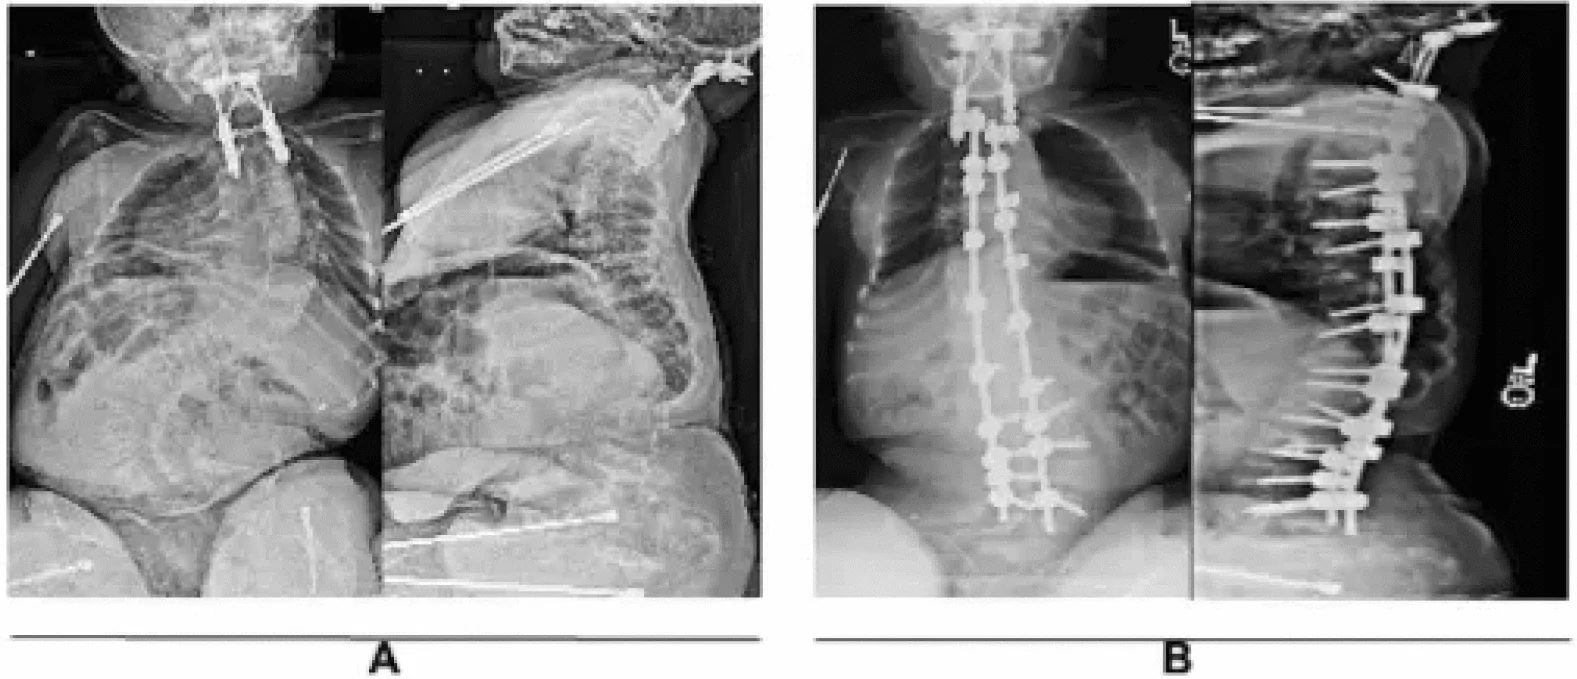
A Preoperative radiographs of a 14-year-old female with type IV osteogenesis imperfecta (OI) and history of prior cervical spinal fusion showing double major curvatures of 112° and 75°. Preoperative measurements included apical vertebral translation (AVT) of 92 mm, thoracic kyphosis of − 38°, thoracolumbar kyphosis of 31°, and lumbar lordosis of 75°. Preoperative SRS-22 total score was 3.0 (pain 2.8, function 3.3, self-image 2.4, mental health 3.4, satisfaction 3.0), CP CHILD total score was 52 (activities of daily living 52, positioning/transferring/mobility 45, comfort and emotions 40, communication and social interaction 55, health 50), and PROMIS T-scores were peer relationships 12, fatigue 25, pain interference 28, and physical function mobility 15. B 2-year postoperative radiographs following posterior spinal fusion with segmental pedicle screw instrumentation. The proximal construct was connected to the existing cervical hardware, while cement augmentation was performed at the distal foundation levels. Radiographic measurements showed correction of major and minor curves to 31° and 28°, respectively, with AVT of 23 mm. Sagittal parameters were corrected to thoracic kyphosis of 40°, thoracolumbar kyphosis of 2°, and lumbar lordosis of 52°. Patient-reported outcomes improved with SRS-22 total score of 3.7 (pain 3.6, function 4.0, self-image 3.4, mental health 3.6, satisfaction 4.5), CP CHILD total score of 67 (activities of daily living 74, positioning/transferring/mobility 58, comfort and emotions 53, communication and social interaction 68, health 80), and PROMIS T-scores of peer relationships 16, fatigue 20, pain interference 17, and physical function mobility 22
